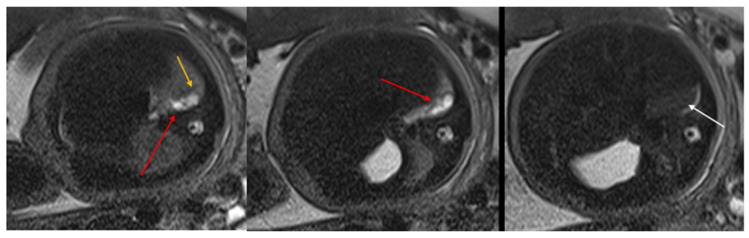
CPAM type II. Axial HASTE T2 MR images show a hyperintense lesion characterized by at least six cystic images (red arrow) of 2–6 mm at the lung base, with extension to the right posterior lung (orange arrow). No compression on the diaphragm or mediastinum. Minimal pleural effusion layer (white arrow).
